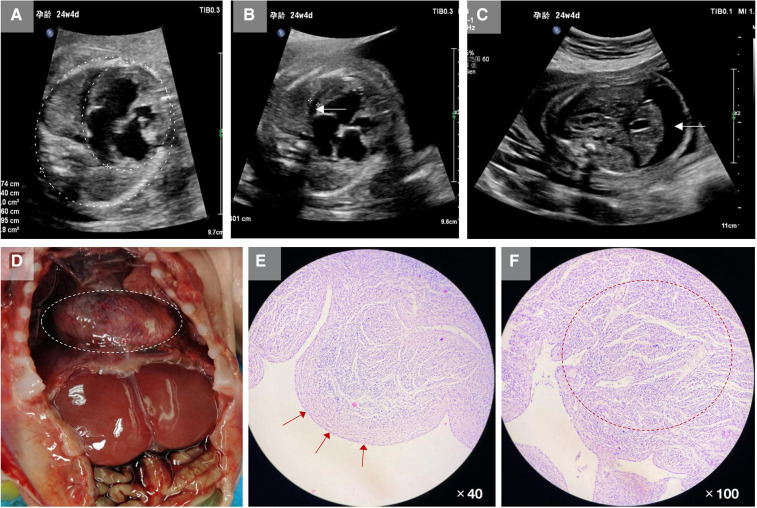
Clinical characteristics of the fetus in this study. (A) Ultrasound showed that the fetal cardiothoracic area ratio was increased, approximately 0.45, and the left atrium and left ventricle sizes were increased substantially. (B) Ultrasound showed hypertrophy of fetal myocardium and free fluid in the pericardial cavity (white arrow). (C) Ultrasound showed a large amount of free fluid in the fetal abdominal cavity and thickened abdominal skin tissue (white arrow). (D) After induced labor, autopsy revealed the heart was considerably enlarged. (E) Pathological sections showed thickening and fibrosis of the endocardial tissue (red arrows). (F) The muscular trabeculae were thick, the sinus recess of the myocardium persisted, the deep depression was staggered, the formation of dense myocardium in the corresponding area was reduced and thin, and the ventricular wall muscle layer remained loose (red circle).
